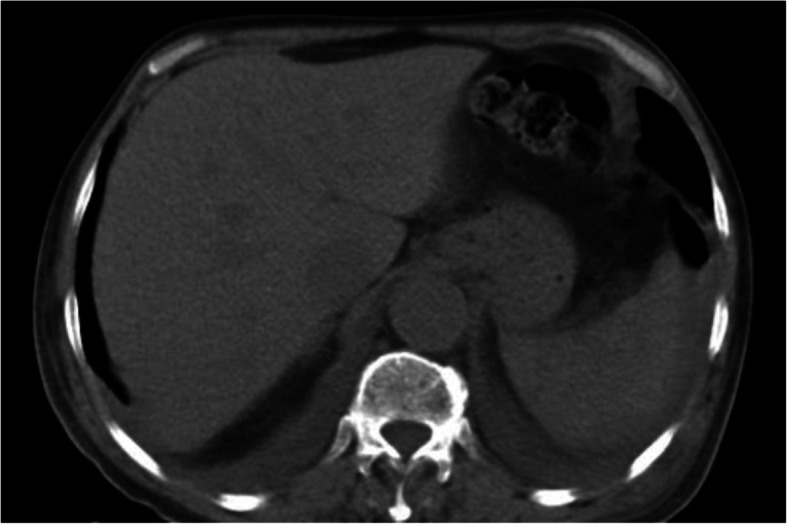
Abdomen view in Secondary CT scan. An extensive fluid collection around the spleen was observed on the CT scan performed for the patient
